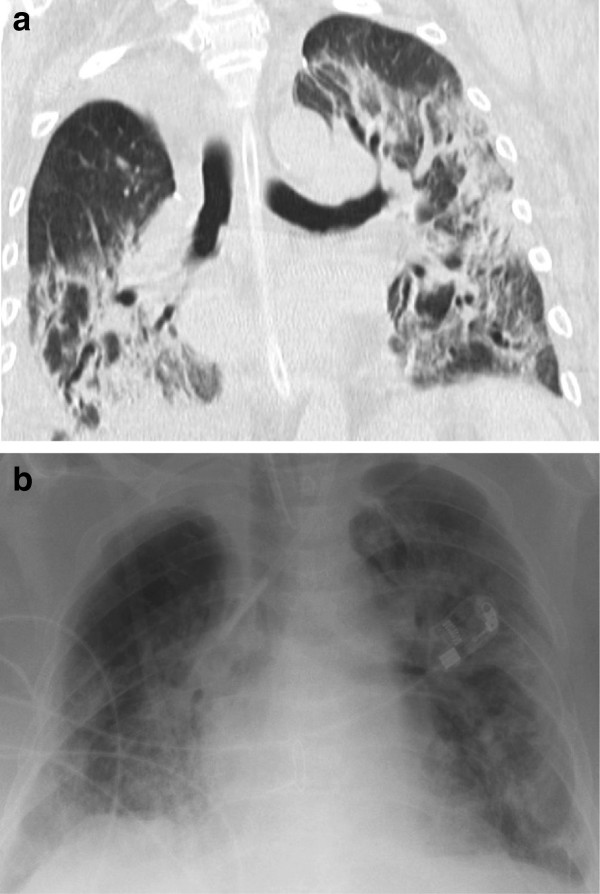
Radiographic findings: computer tomography shows bronchiectasis and consolidation especially in the right lower lobe (a) and increasing bilateral diffuse pulmonary infiltrates in both lungs with ground glass opacities seen in chest x-ray (b).
